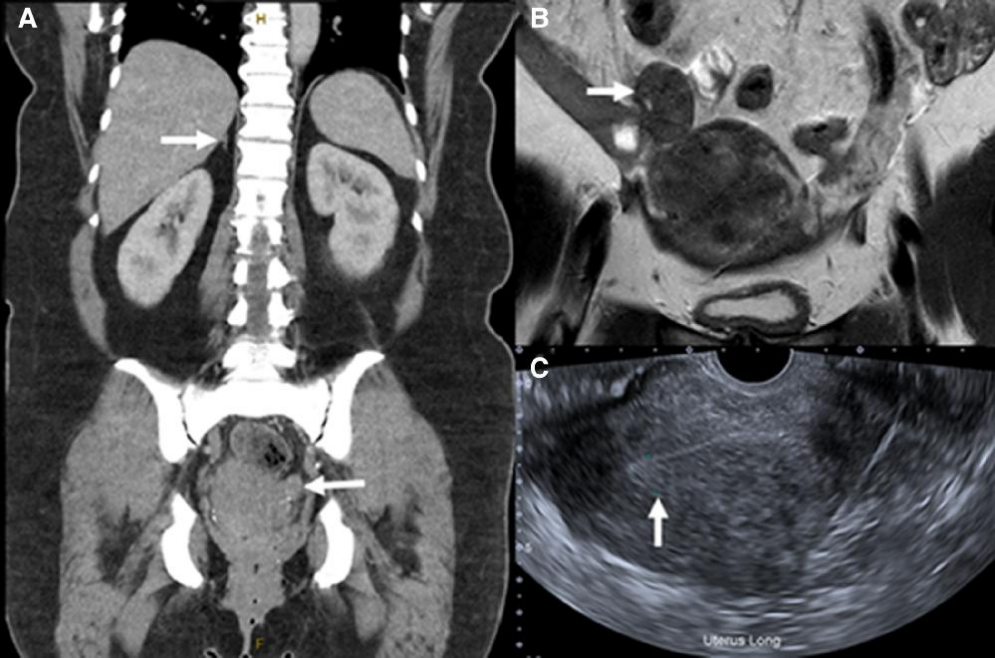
Abdominal A, computed tomography (CT) and B, magnetic resonance imaging (MRI) scans were inconclusive, with no obvious adrenal or ovarian masses. A, The coronal CT view shows the left ovary (arrow) with no masses evident and the right adrenal gland (arrow) with normal contour. B, The coronal MRI view shows the right ovary (arrow) without any identifiable lesion. C, A pelvic ultrasound scan was also inconclusive with no obvious ovarian masses but demonstrated uterine fibroids and endometrial thickening (arrow).
